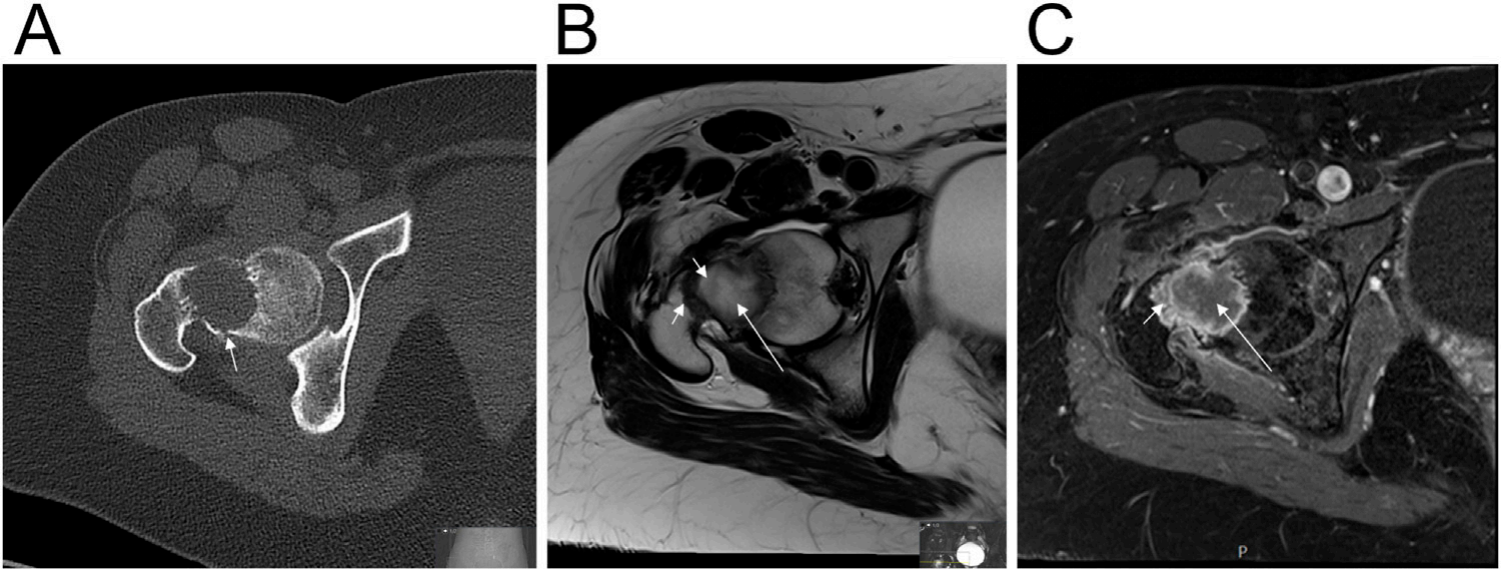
Radiological features of the fibro-osseous tumor. (A) Axial CT image shows a well-defined osteolytic lesion in the femoral neck with a pathological fracture (arrow) and no visible mineralization. (B) Axial T2-weighted MRI of the lesion shows a central part with high signal intensity which may represent water content (long arrow), and a peripheral border as well as intralesional stripes, both with low signal intensity, which may correspond to fibrous content (short arrows). (C) Axial Fat-suppressed contrast-enhanced T1-weighted MRI of the lesion reveals that the central part doesn’t have contrast enhancement which representing water content (long arrow). The peripheral border has contrast enhancement indicating vascularized tissue (short arrow).
